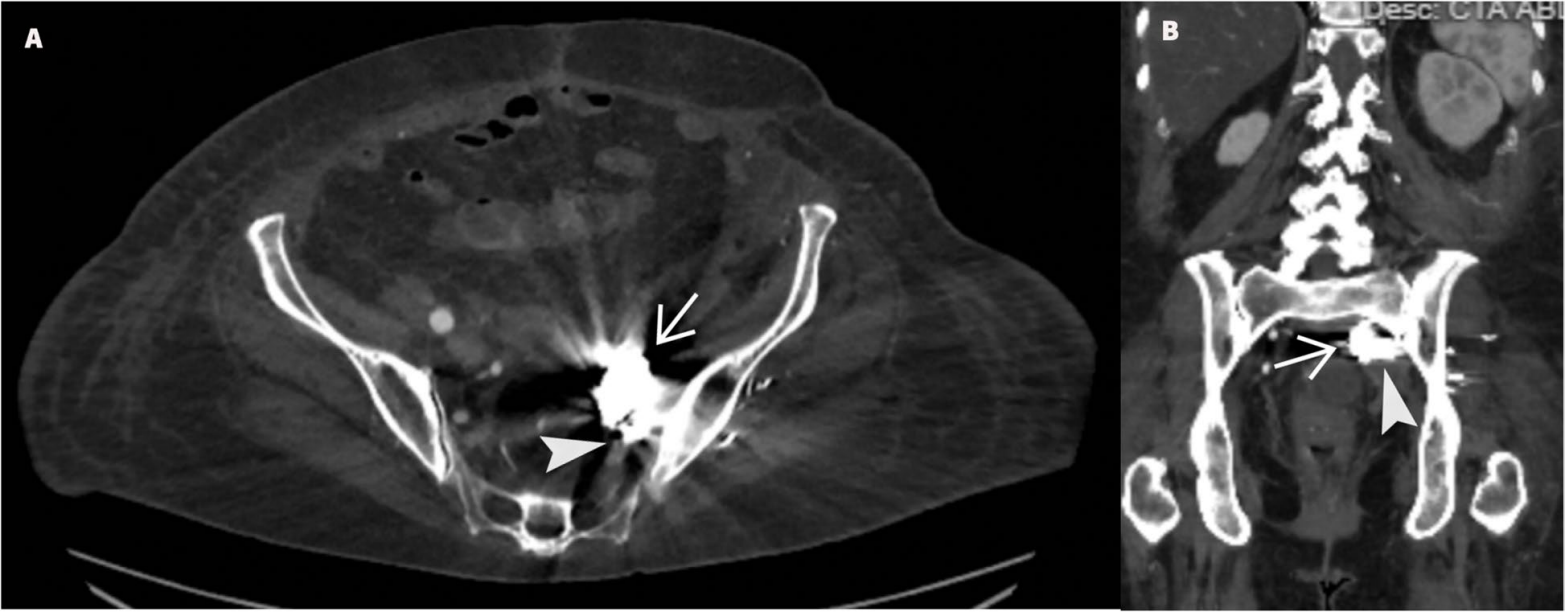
Follow up CT angiogram of the embolized left internal iliac artery mycotic pseudoaneurysm. A) Axial CT image and B) MPR coronal CT image of completely embolized left internal iliac artery mycotic pseudoaneurysm with onyx (arrow) coils (arrowhead) without contrast in the sac
